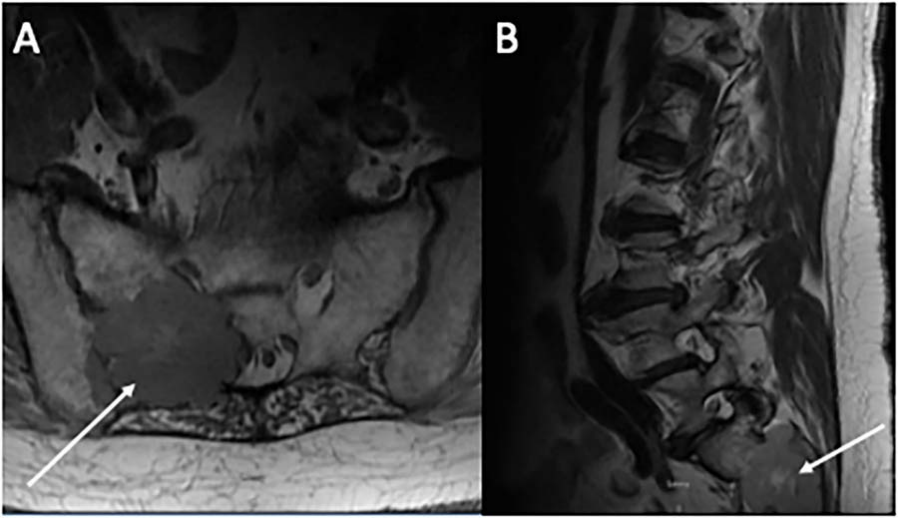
Magnetic resonance imaging of the lumbar spine. (A) Axial and (B) sagittal sections demonstrating an expansive 5 × 4 cm lesion in R sacroiliac joint. CT-guided core biopsy of the mass confirmed metastatic PTC.
